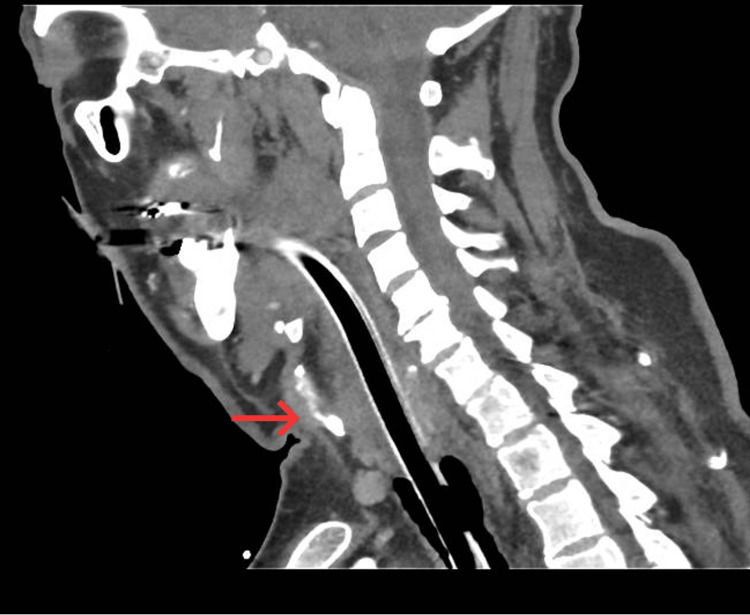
Sagittal CT of the neck demonstrating supraglottic, glottic and subglottic oedema following endotracheal intubation. Red arrows highlight soft-tissue swelling at the supraglottic, glottic and subglottic levels, resulting in airway narrowing.
